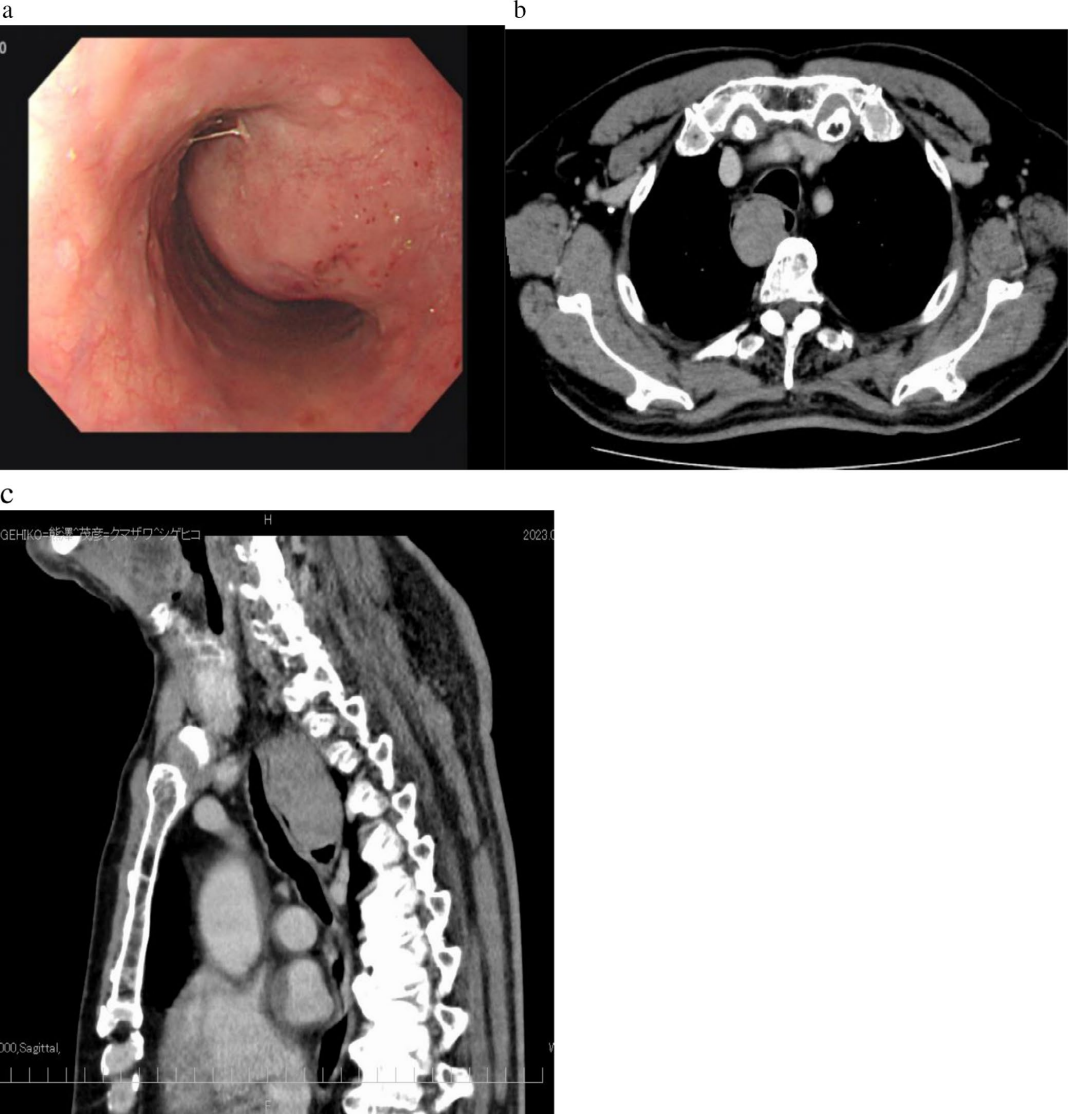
(a) Upper gastrointestinal endoscopy showing a large protruding submucosal lesion, approximately 50 mm in size, located in the upper thoracic esophagus between 21 and 26 cm from the incisor teeth. (b, c) Enhanced CT scans (coronal and axial views) demonstrating a well‐defined tumor with a maximum diameter of 55 mm. The mass is observed primarily in the upper thoracic esophagus, with clear extension into the cervical esophagus. CT showed a well‐defined tumor with a maximum diameter of 55 mm was observed, primarily in the upper thoracic esophagus, extending into the cervical esophagus.
